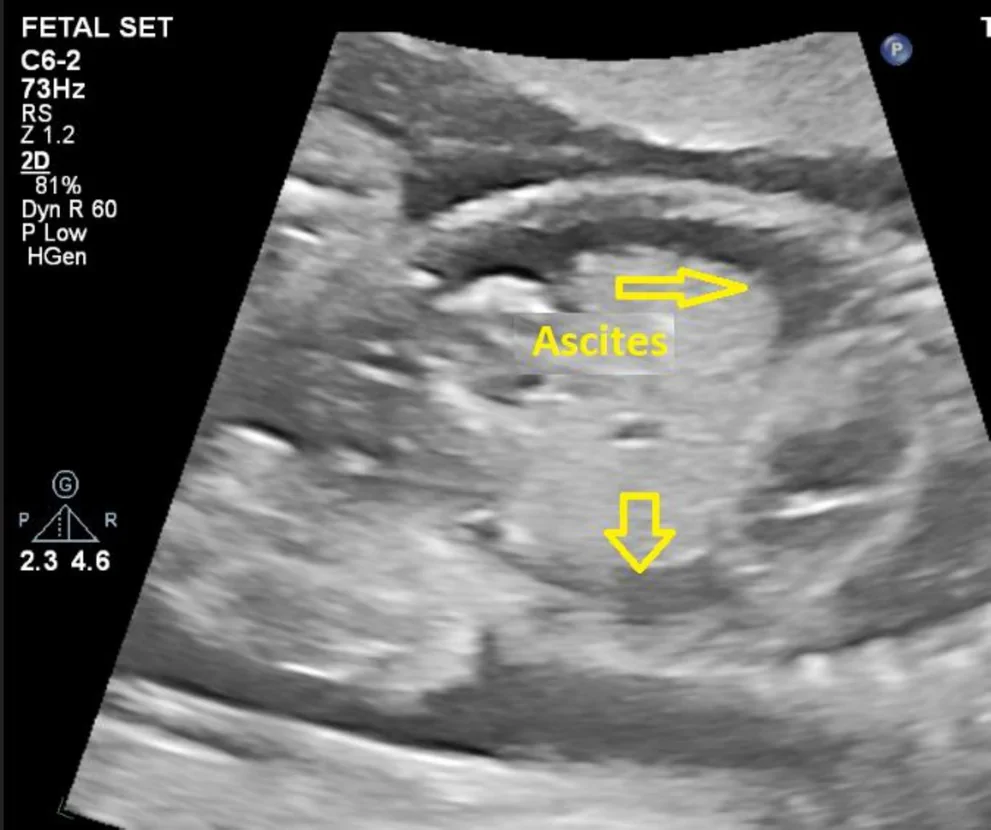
Sagittal sonographic view of the fetus demonstrates marked ascites (yellow arrows), consistent with evolving hydrops fetalis.
